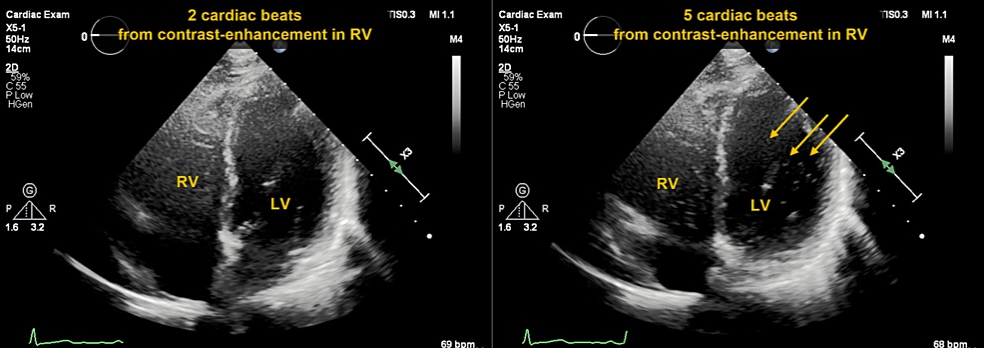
Microbubble-contrasted echocardiography from the apical four-chamber view showed opacifications (arrows) in the left ventricle after five beats from entering the right ventricle.
